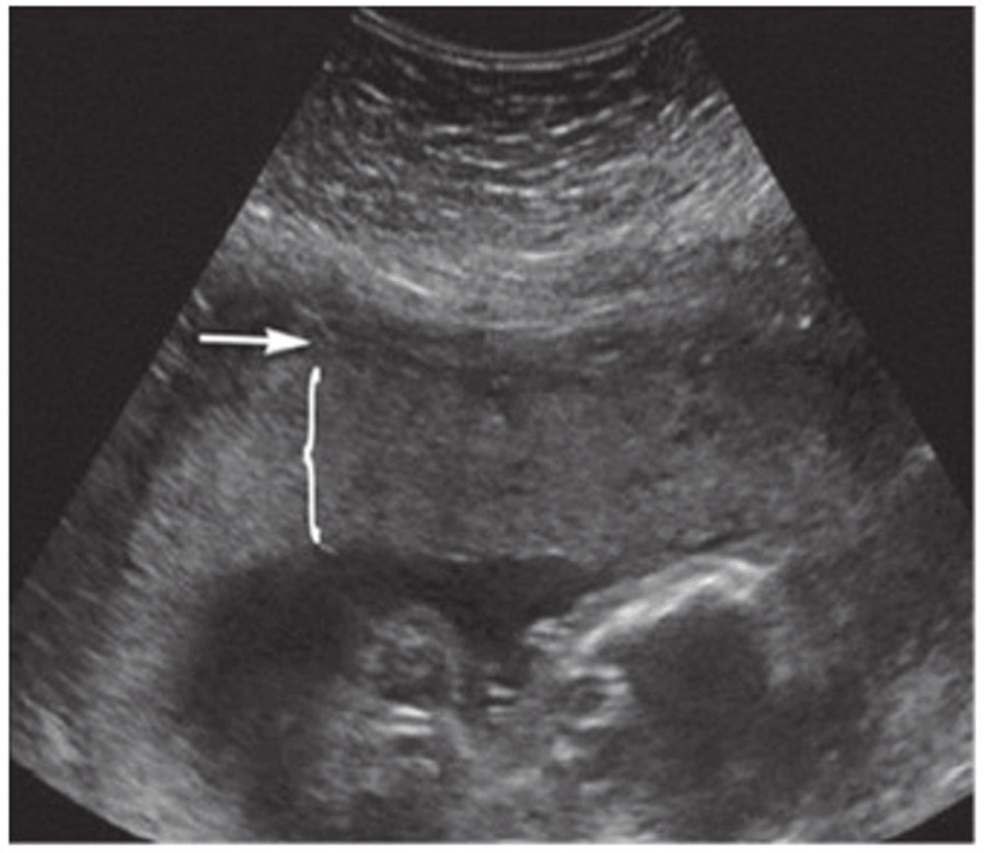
Ultrasound image of a second trimester pregnancy Shows the retroplacental complex on the transabdominal grayscale USG and how measurements of placental thickness were taken in this study. USG: ultrasonography
